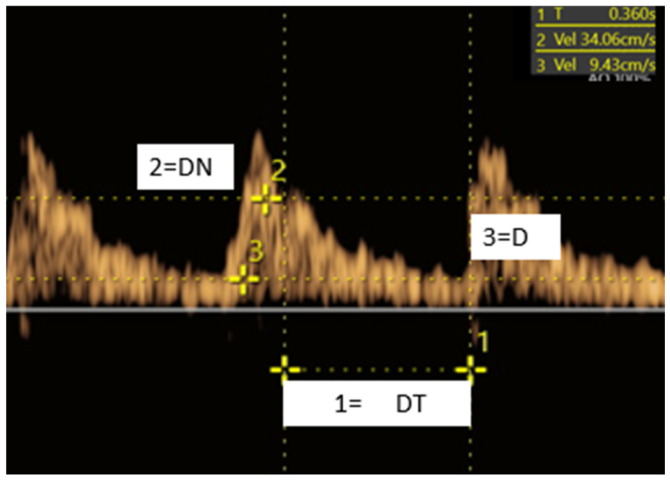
Sonographic assessment method of the middle cerebral artery (MCA) diastolic deceleration area (DDA). 1 = DT, deceleration time (corresponding to T on the device display); 2 = DN, dicrotic notch (corresponding to Vel2 on the device display); 3 = D, end-diastolic velocity (corresponding to Vel3 on the device display).
